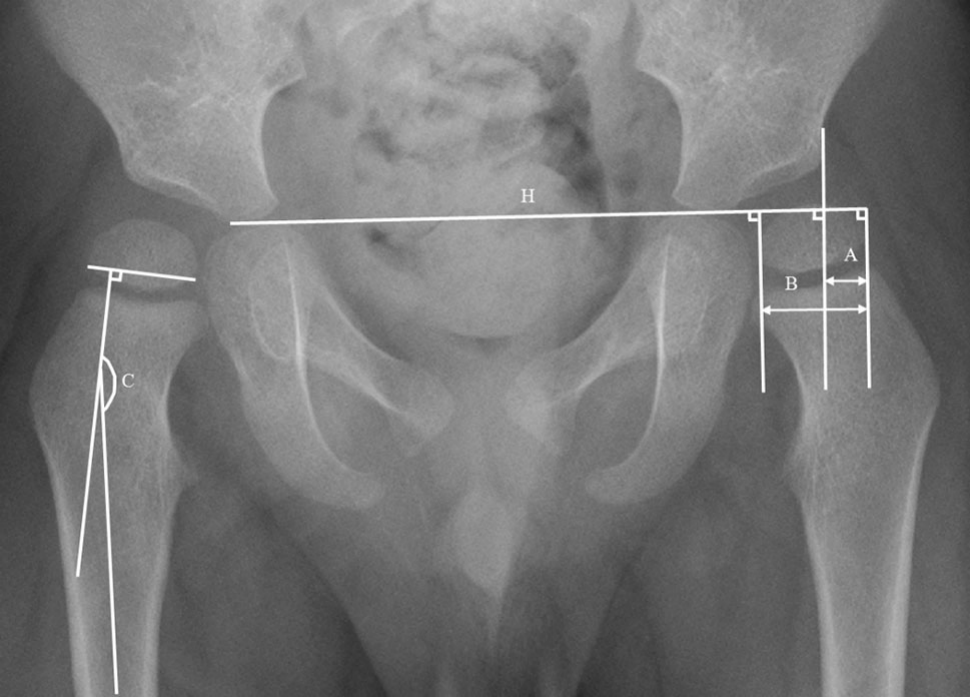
Anteroposterior (AP) radiograph of the pelvis. Right hip: head-shaft angle (*C*) by measuring the medial angle between a line perpendicular to the proximal femoral epiphysis and a line through the middle of the femoral shaft. Left hip: the migration percentage (MP) is measured by a Hilgenreiner’s line (*H*) and three perpendicular lines. The MP is measured by *A*/*B* × 100 %
